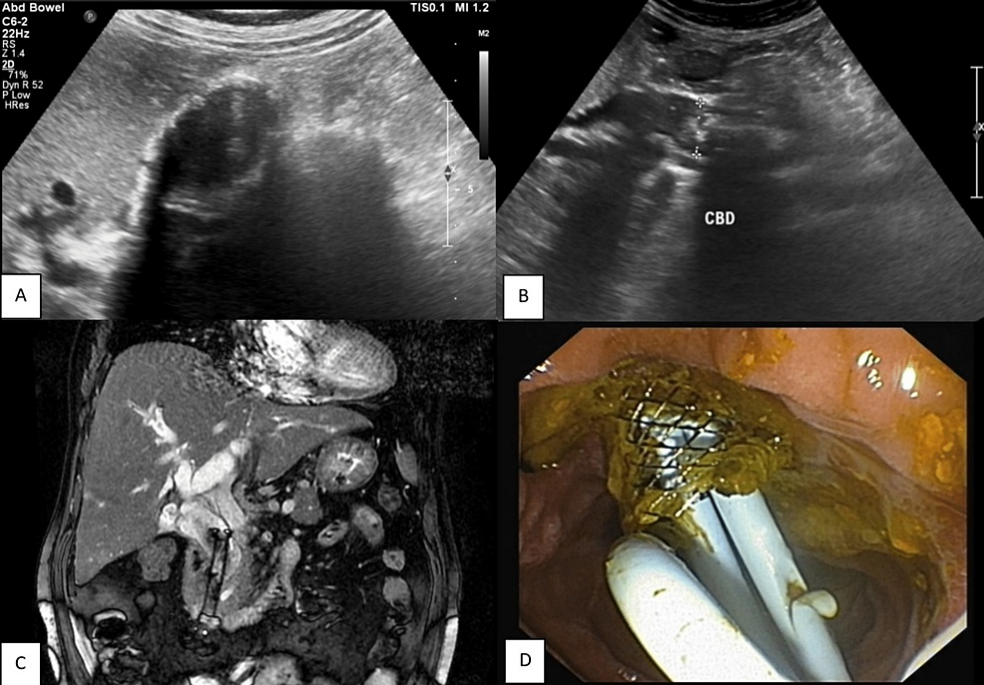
Blocked self-expanding metallic stent with porcelain GB. (A) Transabdominal ultrasonography shows calcified and thickened gallbladder wall; (B) Transabdominal USG shows dilated common bile duct with stent in situ and non-homogenous echogenic material; (C) Coronal T2 SSFSE shows cholelithiasis with porcelain GB and bilobar intrahepatic biliary radicle dilatation with common bile duct stent in situ; (D) Removal of blocked stent GB: gallbladder; SSFSE: single-shot fast spin echo
